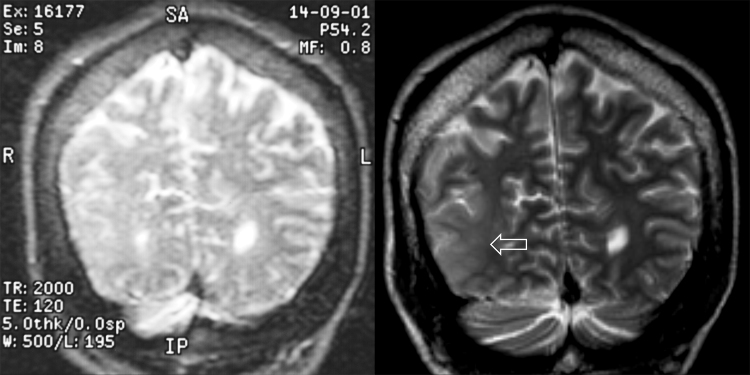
Refractory partial epilepsy with right inferior parietal malformation of cortical development. Patient with refractory partial epilepsy and learning disability. Imaging in 2001 at 1.5 T was reported as normal (A) but subsequent imaging in 2004 at 3 T revealed an extensive right inferior parietal malformation of cortical development (B). Images are coronal T2-weighted images at the posterior edge of the occipital horns.
